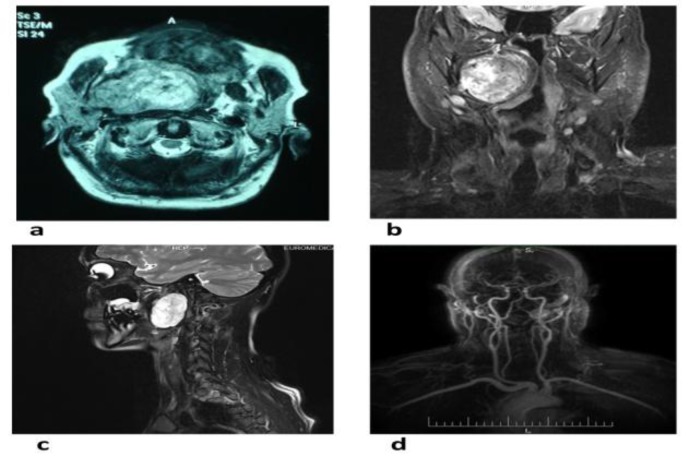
Computed tomography and magnetic resonance imaging scans in axial (a), coronal (b), and sagittal (c) planes demonstrating giant parapharyngeal space tumors lesions laid in the prestyloid compartment, (d) implementation of magnetic resonance angiography (Patient 6) due to superomedial tumor localization that raised concern about its relationship to adjacent vital vascular structures
